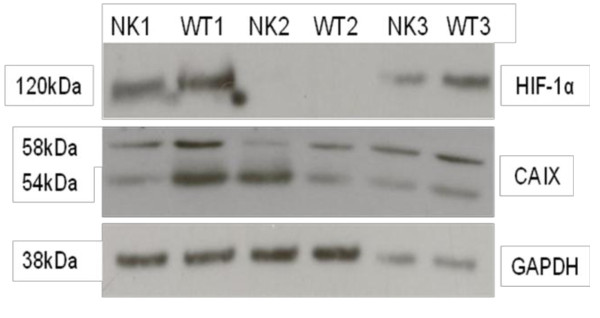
Protein expression in cell lysates from matched-paired NK and WT samples as detected by western blot analysis. Figure 2a. HIF-1α (upper panel) and CA IX (middle panel) and the lower panel indicates even protein loading as evidenced by detection of the GAPDH control from the same extract. GAPDH is visible as a band of approximately 38 kDa. Upper panel: A single band at 120 kDa indicates full length HIF-1α protein detected in two post-CT samples (pairs 1 and 3). HIF-1α is higher in WTs than in matched-paired NKs. Middle panel: CA IX expression, indicated by two bands of 54 kDa and 58 kDa, is higher in two out of three WTs compared to their corresponding NKs.
